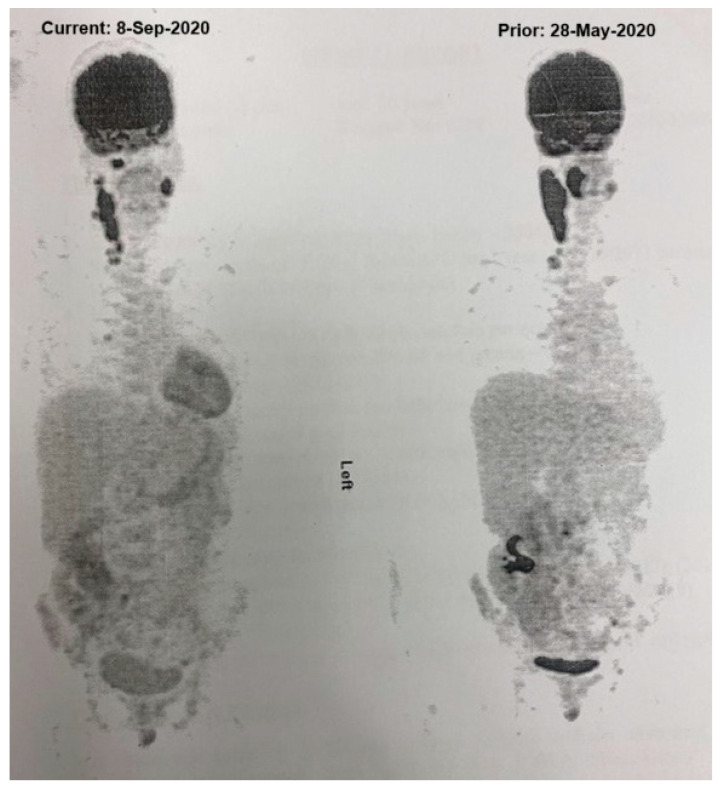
PET Scan Findings Indicating Disease Progression in PTLD; PET scan performed on 13 September 2020, showing evidence of disease progression with the appearance of a new hypermetabolic right retropharyngeal lymph node (SUV max 13.5) and an interval increase in metabolic activity in cervical and pelvic lymph nodes, consistent with progressive PTLD. This image reflects the initial presentation and the first relapse.
